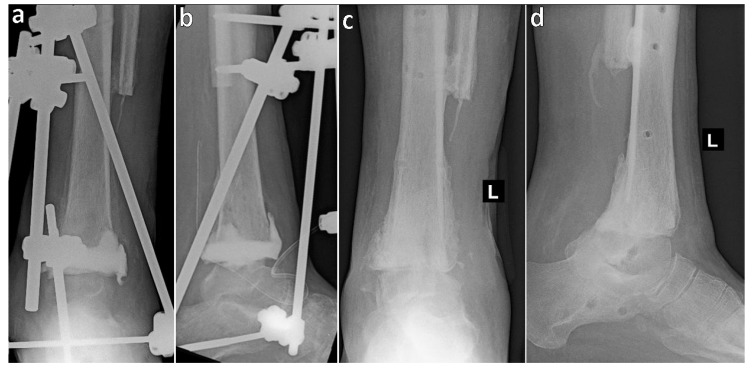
Radiographs of a patient with ankle osteomyelitis: (a,b) after resection of the tibial and talar articular surfaces with insertion of an antibiotic-loaded cement spacer and external fixation; (c,d) final status following tibiotalar arthrodesis with external fixation and autograft spongioplasty, with subsequent removal of the fixator.
